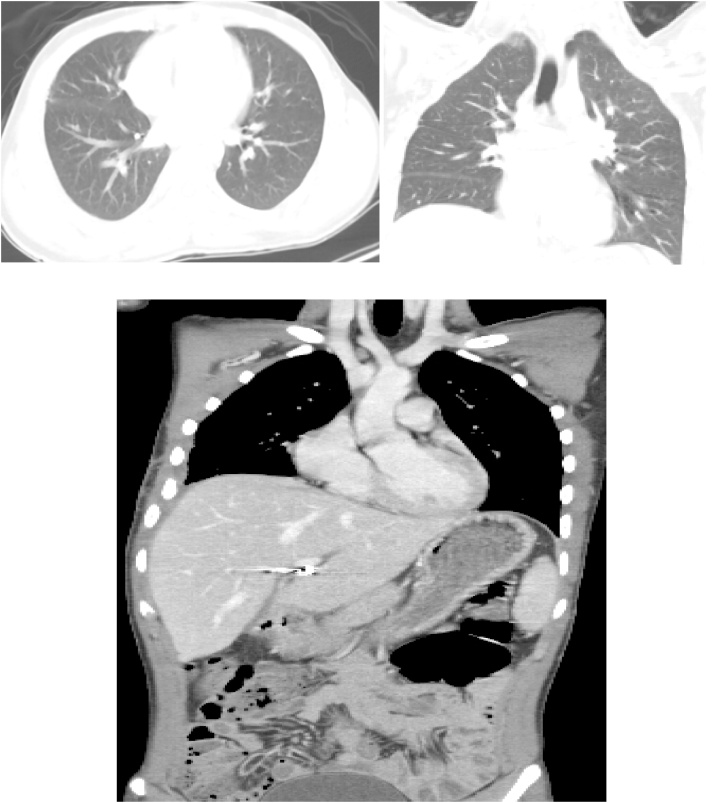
CT chest & Abdomen show normal looking both lung and liver parenchyma with no evidence of recurrence of the IHPA.
